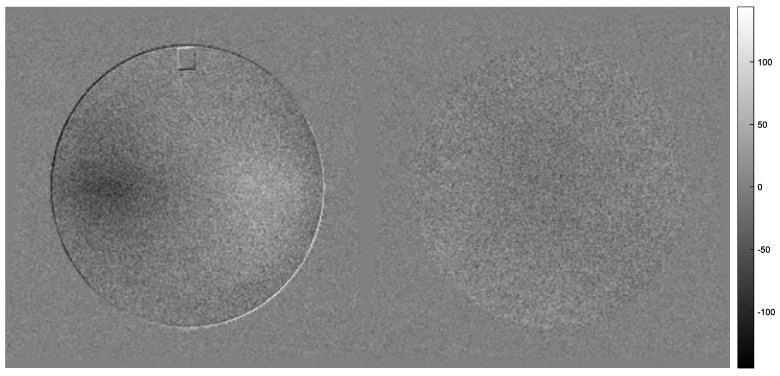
An example of the image that results when subtracting slice #6 from slice #7 acquired in the same acquisition is shown in the left. An example of the image that results when slice #7 acquired in series B is subtracted from slice #7 acquired in series A is shown in the right. Both examples are for sequences acquired with the GE Genesis Signa 1T MRI system.
